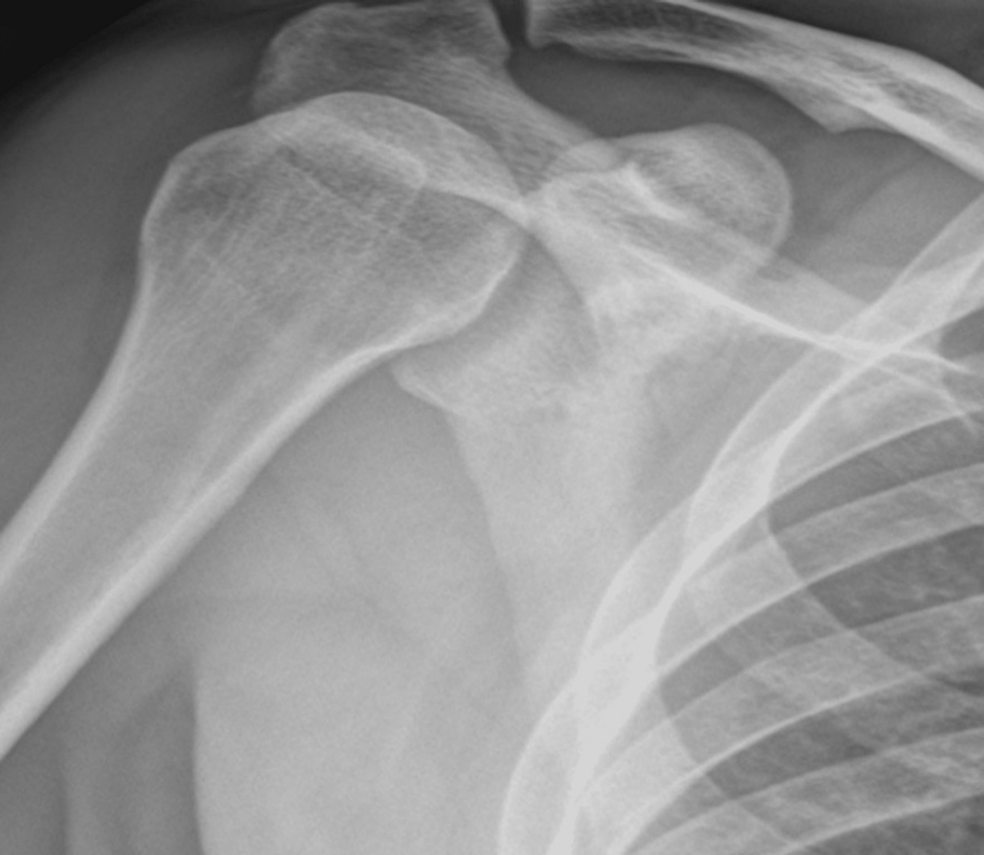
Anteroposterior X-ray of the right shoulder after close reduction by two-step technique maneuver
